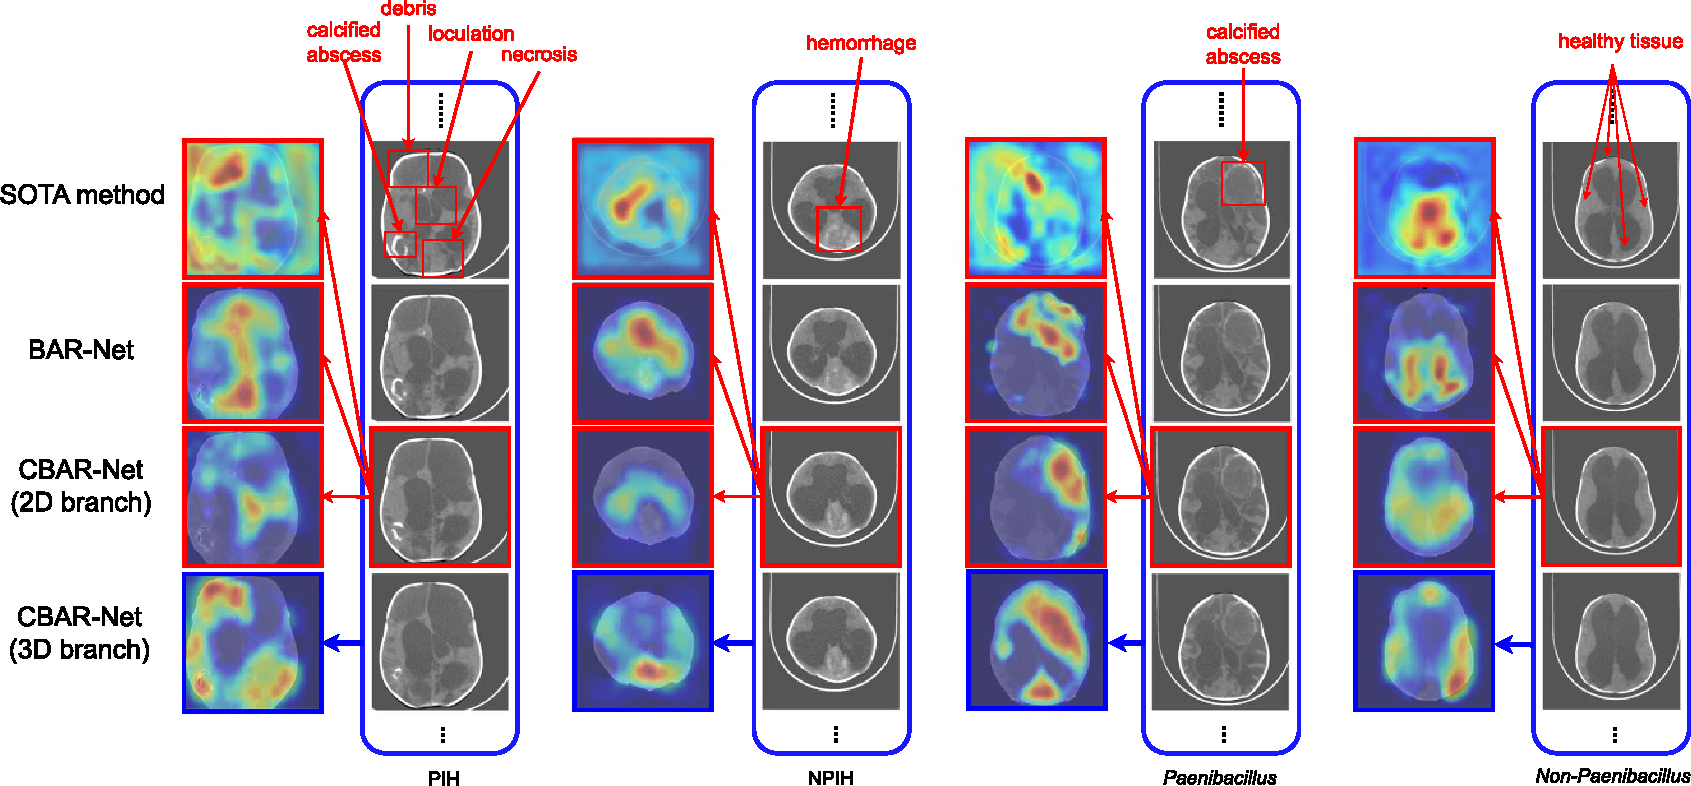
The CAMs (upscaled for visualization) of the best performing SOTA method [10], BAR-Net, 2D branch and 3D branch of CBAR-Net are shown. The CT image stack shown in each column are from examples of PIH, NPIH, *Paenibacillus*, and non-*Paenibacillus* cases from left to right respectively.
